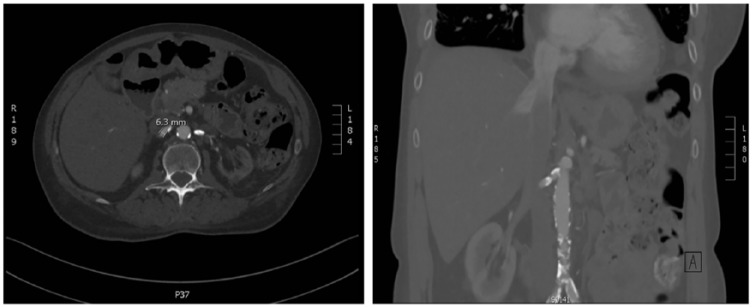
Horizontal and coronal views of abdominal computed tomography angiogram
